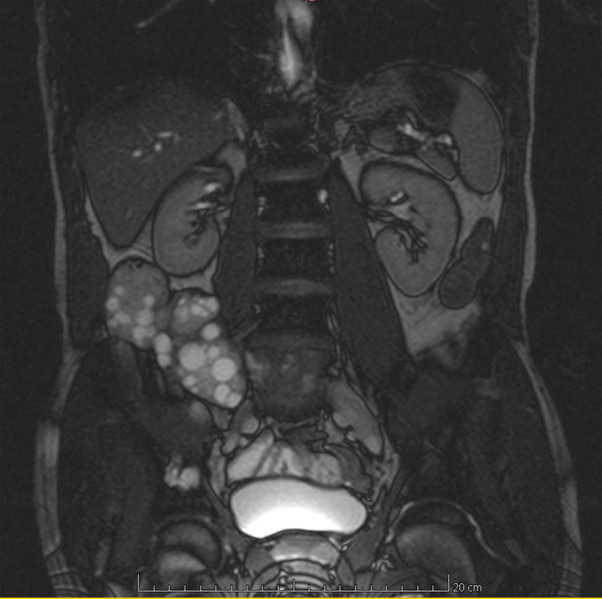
Demonstration of a retroperitoneal multilocular hypointense cystic lesion extending from the right ileopsoas muscle to the subhepatic area on coronal T2-weighted abdominal magnetic resonance image.
